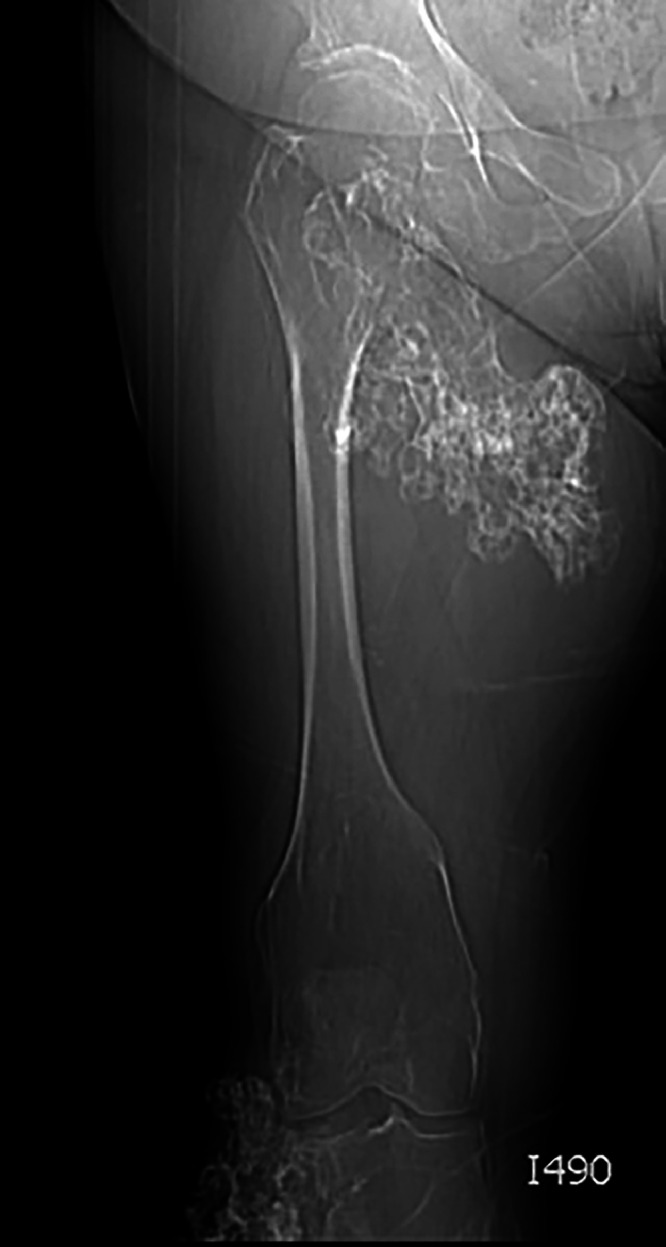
Right leg plain film, anteroposterior projection. It showed an exostosis originating from the femoral neck and involving the metaphysis. The lesion was in continuity with the medullary cavity. Margins were irregular. There were no signs of fracture. Another exophytic exostosis originated from the lateral side of the proximal tibial epiphysis.
